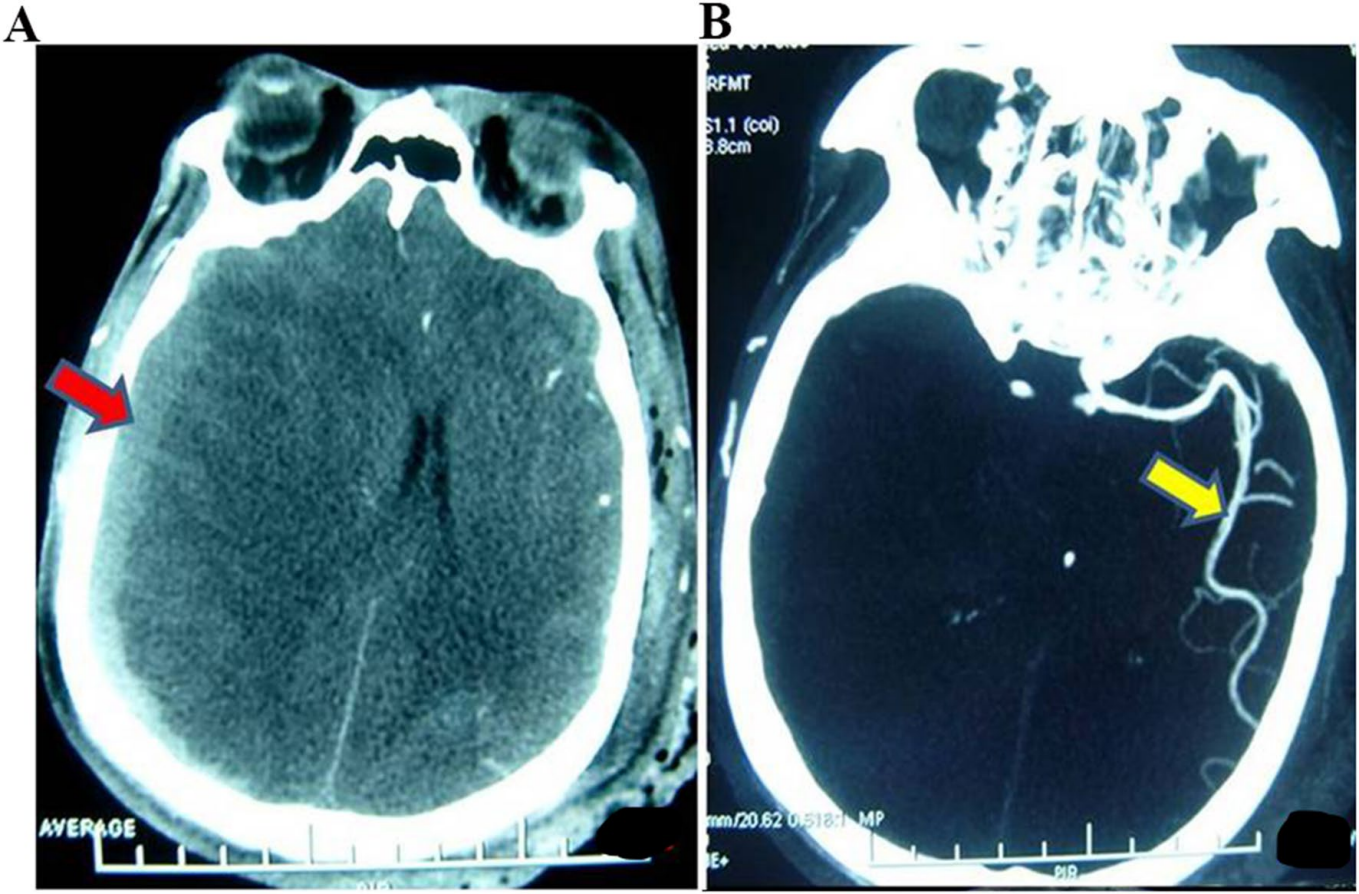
LAO of CTA. A case of a severe TBI patient with GCS 4. (A) CT indicates acute subdural hematoma. (B) CTA indicated that the right ICA and MCA were occluded.
